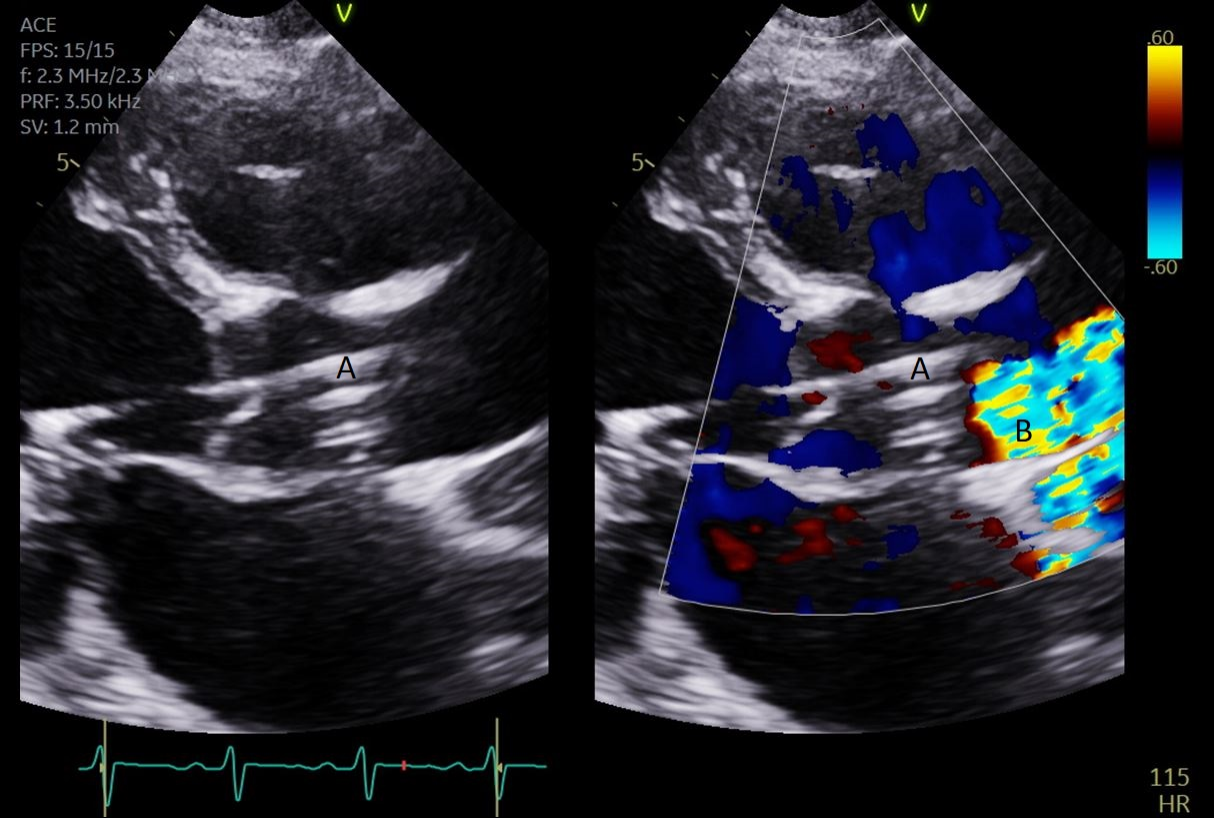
A parasternal long axis view on a transthoracic echocardiogram
